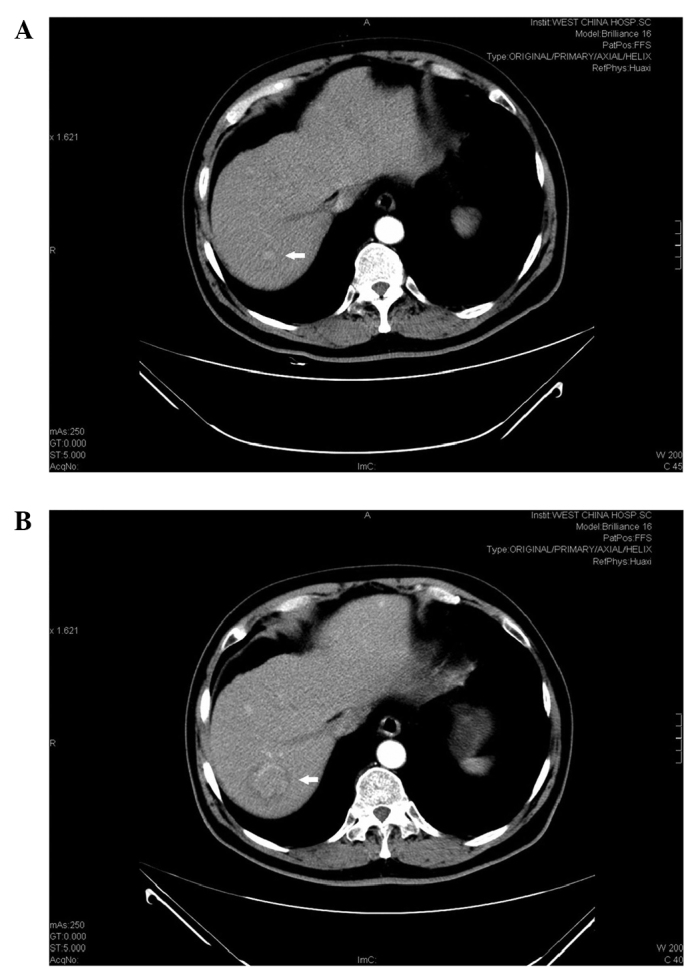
(A) The 0.9-cm tissue mass of unknown malignancy: A contrast computed tomography scan on December 3, 2010, showed a mildly-enhanced lesion in the right lobe of the liver when it was discovered for the first time. (B) The mass of 3 cm in diameter was confirmed as hepatic carcinoma by computed tomography on December 20, 2011.
